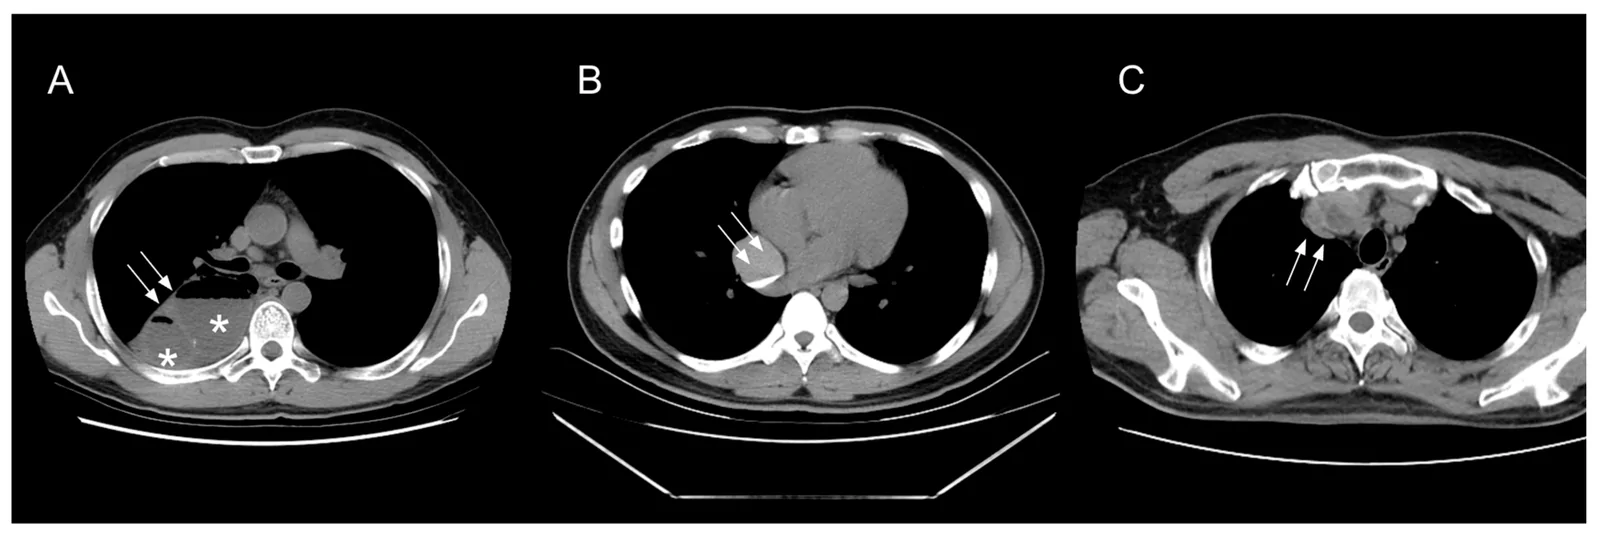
Representative preoperative computed tomography (CT) features of bronchogenic cysts. (A) Lobulated cyst margin (paired arrows) with an internal air–fluid level (asterisks) and dependent fluid layering. (B) Rim calcification (paired arrows). (C) Heterogeneous attenuation manifesting as areas with higher and lower densities (paired arrows) throughout the lesion.
